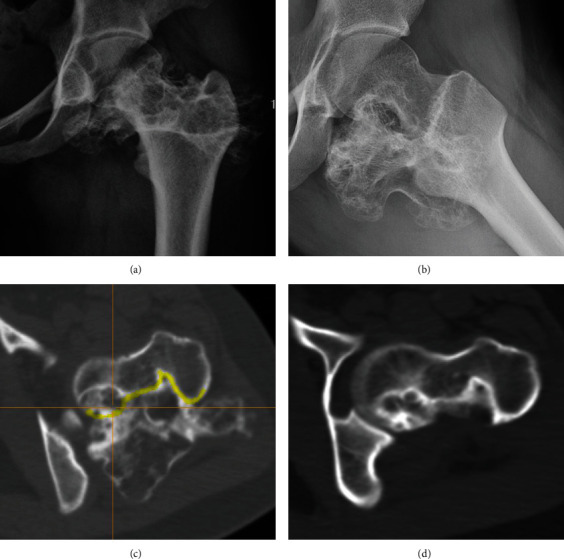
Imaging studies of patient 4. (a) AP and (b) lateral preoperative radiographs. (c) Axial CT with resection plan marked in yellow. (d) Postoperative axial CT.
